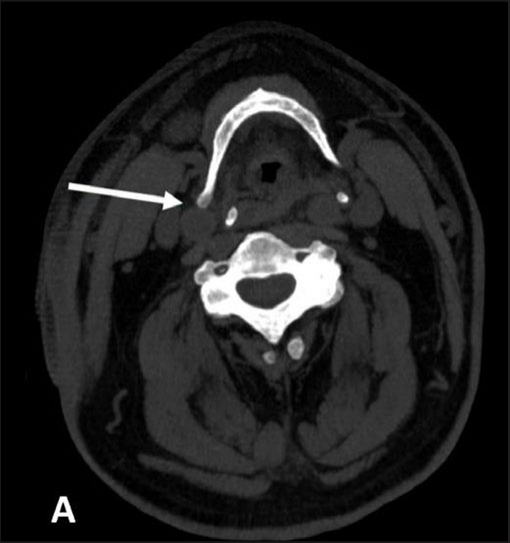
Reformatted non-enhanced oblique axial CT-scan image of the neck (soft tissue window) showing right posterior tilting of hyoid bone with its right greater horn (arrow) abutting the anterior wall of the distal right common carotid artery.
